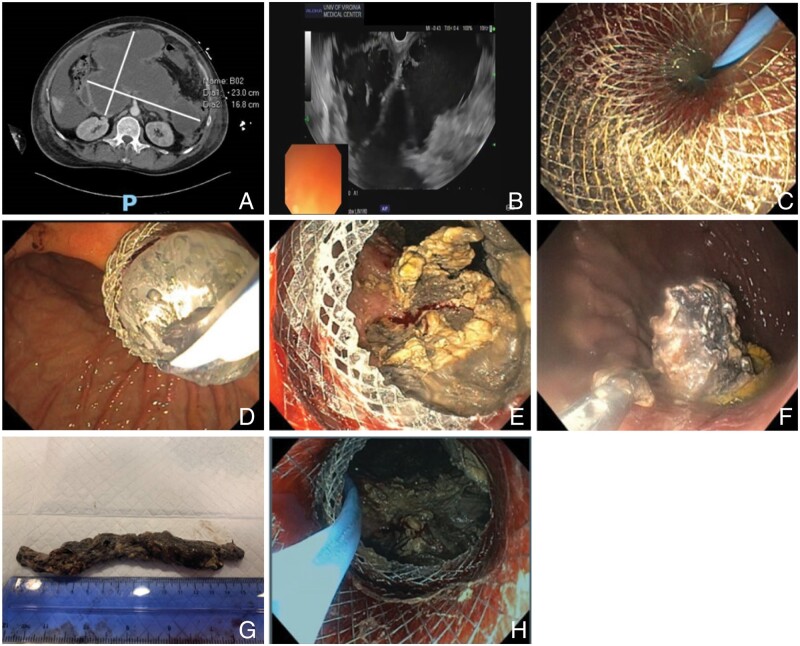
Endoscopic ultrasound (EUS)-directed drainage of pancreatic fluid collections. (A) Axial computed tomography of a pancreatic fluid collection. (B) EUS allows direct visualization of the pancreatic fluid collection. A 19-gauge needle is used to access the cyst and a guide wire is placed in the cavity. (C) The distal end of the lumen-apposing metal stents (LAMS) is placed in the fluid collection using an electrocautery-enhanced delivery system. Next, the proximal/enteral end of the stent is deployed within the echoendoscope and the scope is gradually withdrawn to allow deployment within the stomach or duodenum. (D) Depending on the location of the LAMS and the need for necrosectomy, the size can range from 10 to 20 mm. The tract can then be dilated over the wire with a through-the-scope balloon to allow increased drainage, direct access, and necrosectomy if needed. (E) Typically, the echoendoscope is removed, a gastroscope is introduced, and the cavity can be directly accessed. (F) Various instruments can be used, including snares, baskets, and nets, to debride the cavity. (G) Necrotic pancreas can be systematically removed from the walled-off collection. (H) Double-pigtail plastic stents are placed within the lumen of the LAMS to facilitate drainage and theoretically decrease risk of bleeding and perforation as the cavity contracts.
